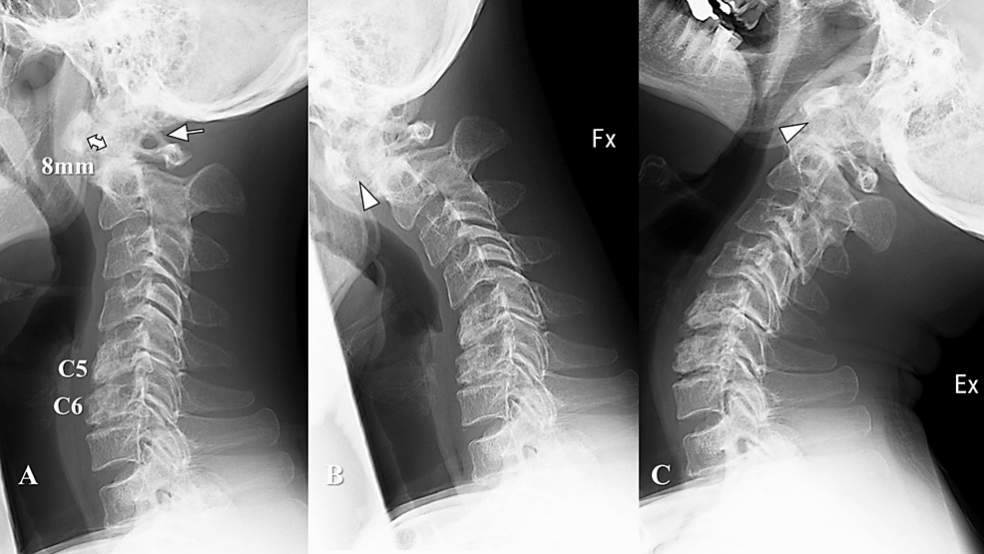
Radiography images at the initial visit. A. Lateral plain radiography in standing position revealed atlantoaxial subluxation with an 8-mm atlantodental distance (double arrow) and ponticulus postics (arrow). Slight spondylotic changes were further observed at the C5/6 and C6/7 spinal levels. B and C. Horizontal atlantoaxial instability during neck flexion (B) and extension (C) was observed (arrowheads).
